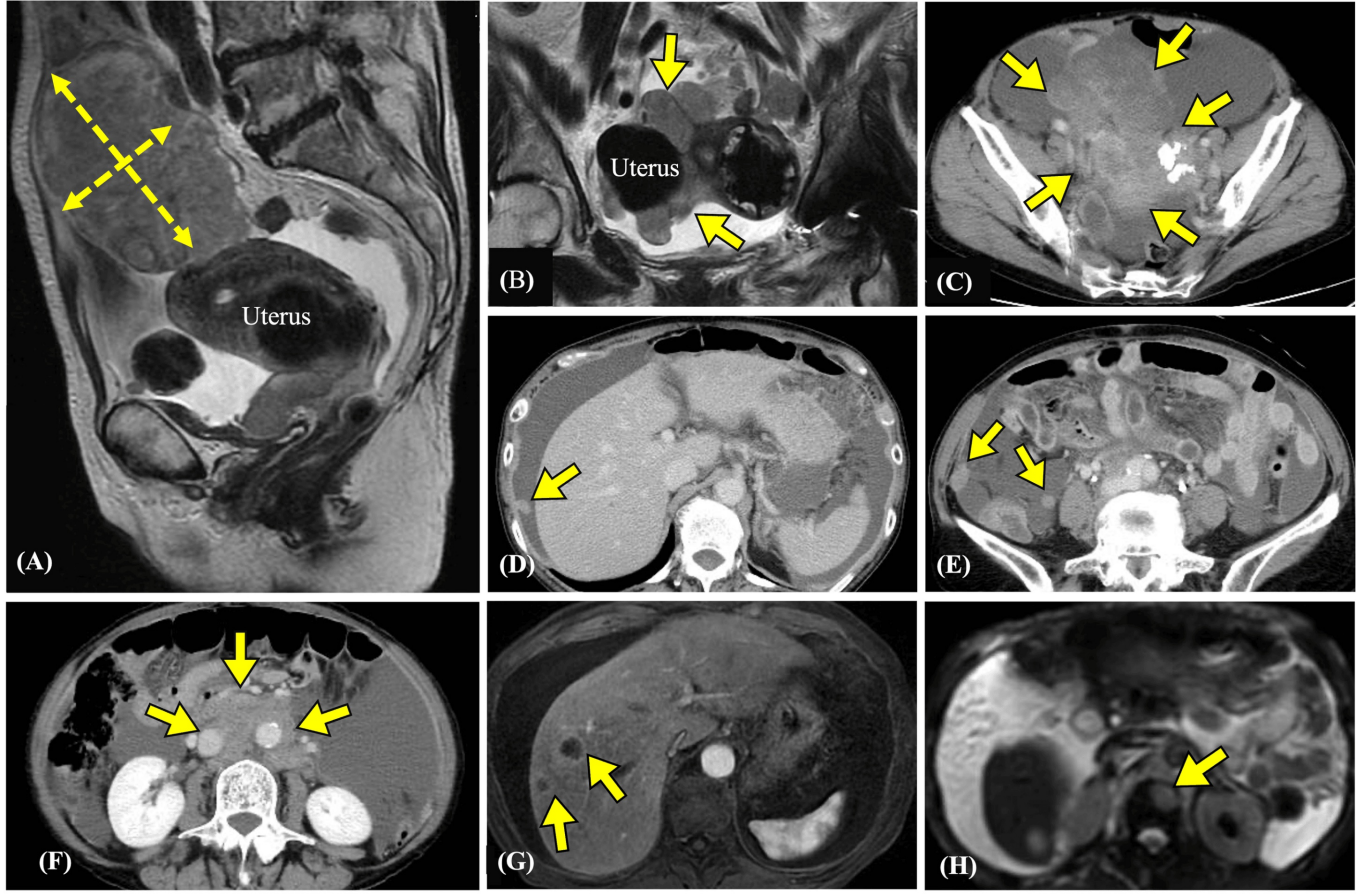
Imaging findings at initial presentation (A) MRI sagittal view showing a solid mass in the pelvis (12 × 7.8 cm). (B) MRI coronal view showing a solid mass around the uterus. (C) CT image showing a solid mass in the right adnexa and uterus. (D, E) CT image showing peritoneal dissemination. (F) CT image showing para-aortic lymphadenopathy. (G) MRI image showing multiple liver metastases. (H) MRI image showing vertebral bone metastases. CT: computed tomography, MRI: magnetic resonance imaging
